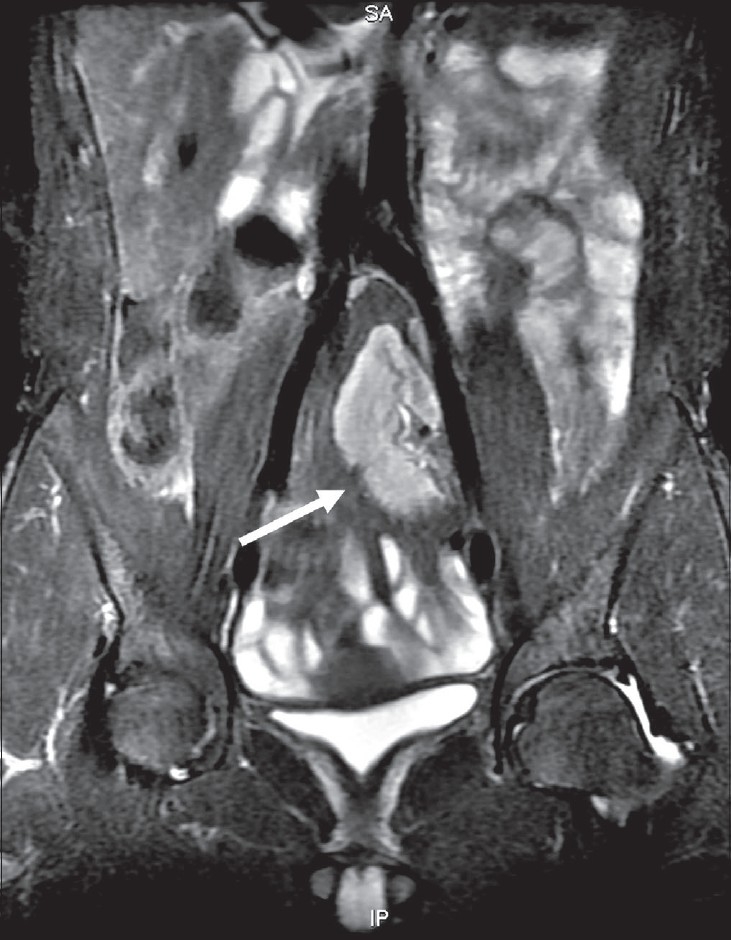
MRI of the lower abdomen and pelvis (coronal fat-suppressed image) shows an ectopic pelvic location of the kidney (arrow). The prostate gland is not visualized
